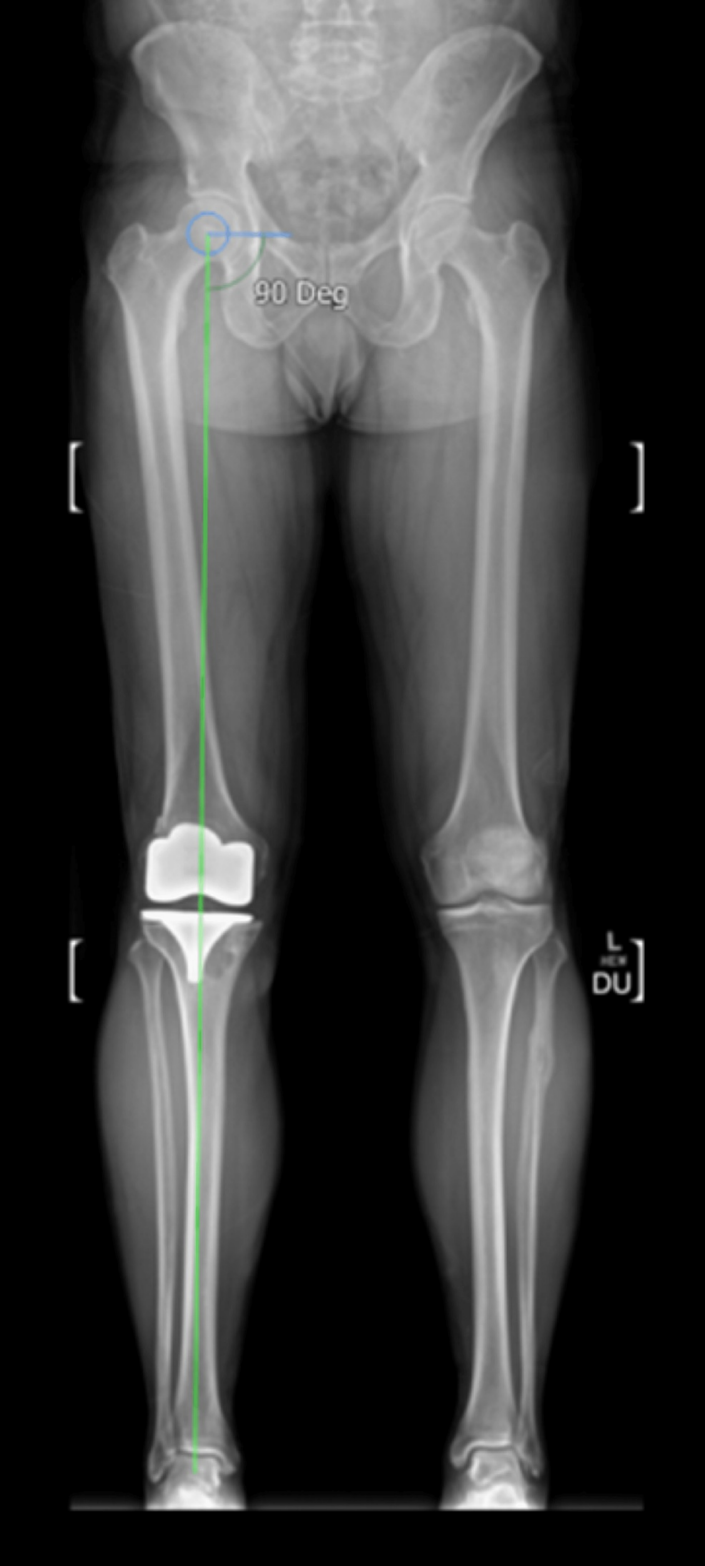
Long leg alignment radiograph before planning revision total knee replacement. The mechanical axis is depicted by the green line drawn from the centre of the femoral head to the centre of the ankle joint.
